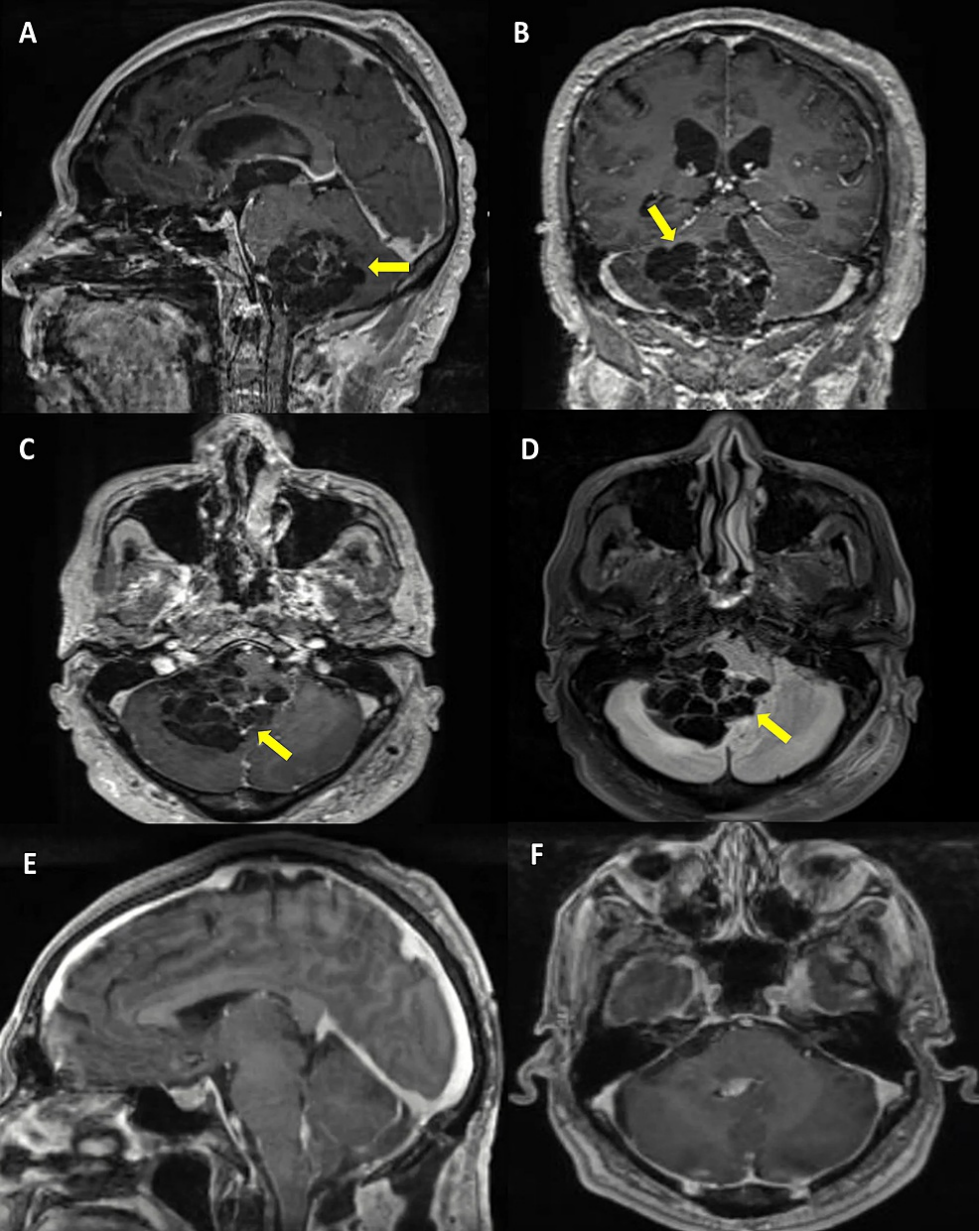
MRI images. (A) Sagittal, (B) coronal, and (C) axial postcontrast T1-weighted images demonstrated an intra-axial multilobulated hypointense mass posterior to the pontomedullary junction that does not enhance and appears to be extending into the fourth ventricle causing expansion on the rostral portion of this compartment (yellow arrows). (D) Fluid-attenuated inversion recovery (FLAIR) sequence in the same patient showed that the cyst fluid is hypointense (yellow arrow). No evident solid component was observed. Postoperative (E) sagittal and (F) axial T1-weighted with contrast images showing gross total resection of the mass.
